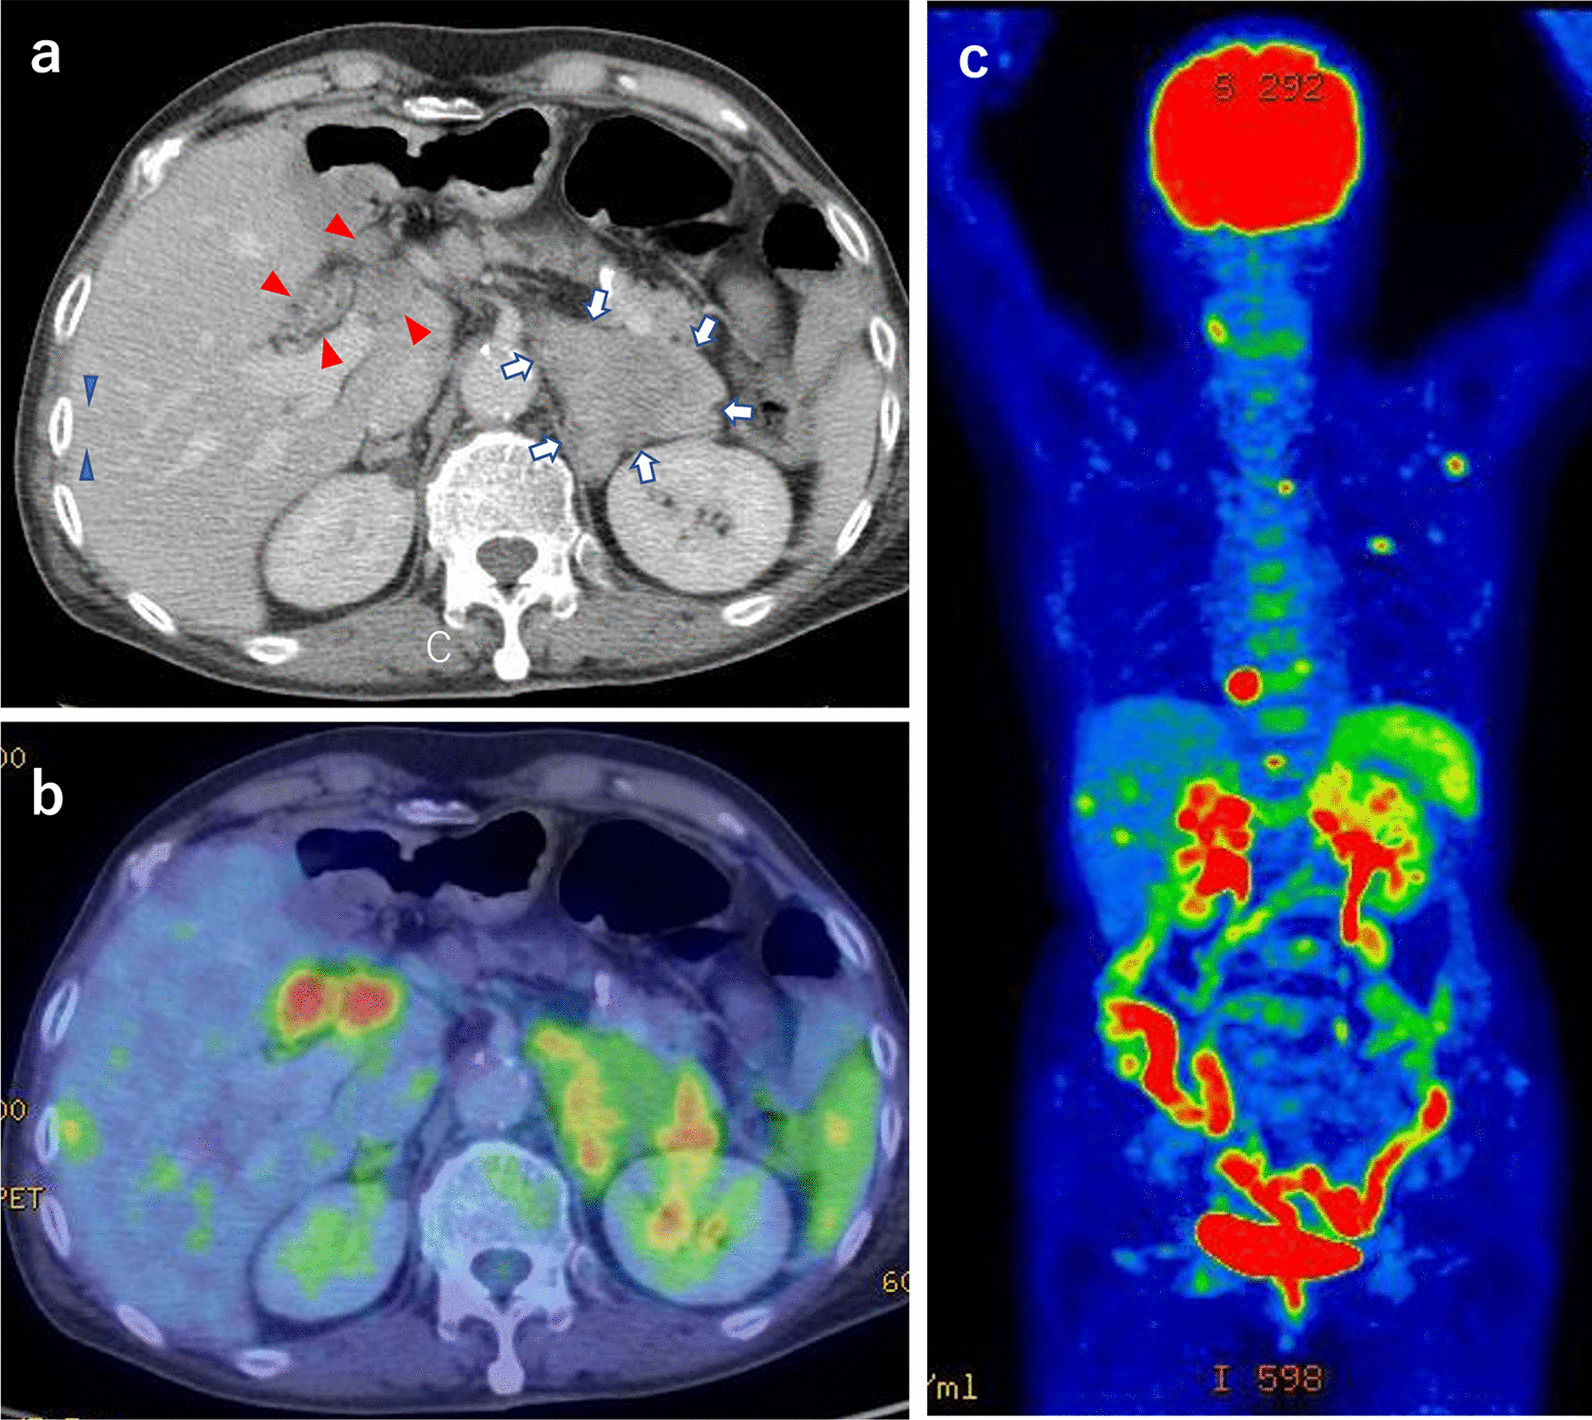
Imaging findings of the patient. a Contrast-enhanced computed tomography images. The image shows a mass in the adrenal gland and the enlarged lymph nodes and liver lesions. Arrows indicate the mass in the adrenal gland. Red and blue arrowheads indicate the enlarged lymph nodes and liver lesions, respectively. b, c Positron-emission tomography. Positron-emission tomography shows fluorodeoxyglucose F18 accumulation in the left adrenal gland; lymph nodes around the renal artery and common hepatic artery; and multiple nodules in the right lobe of the liver, left scapula, left sixth rib, and left lateral process of the ninth thoracic vertebra
